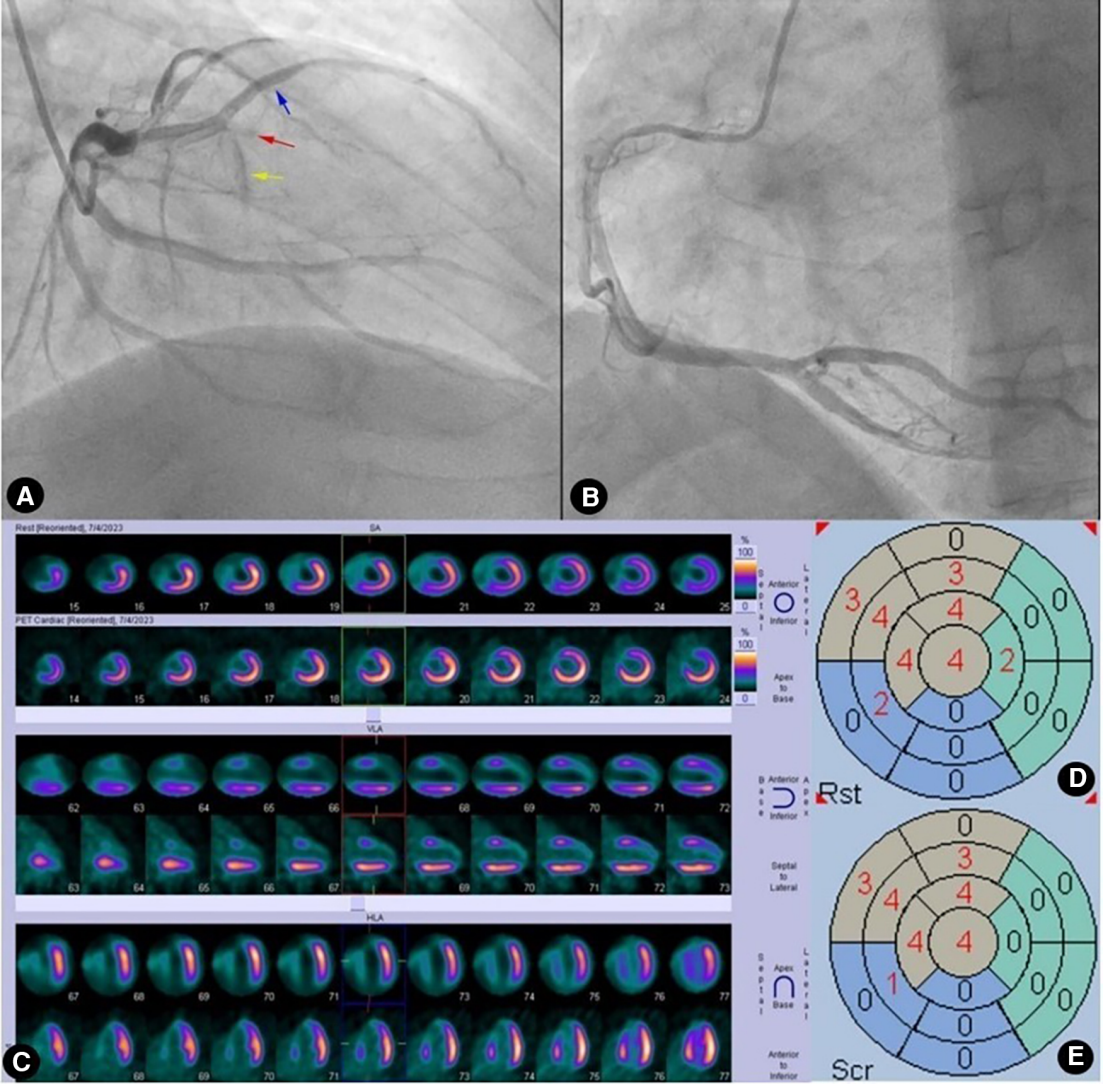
Case 1: male, 60 years old. (A) Coronary angiography showed that the left anterior descending artery (LAD) was completely occluded (red arrow) after emitting a diagonal branch (blue arrow) and septal branches (yellow arrow). (B) Coronary angiography showed no evidence of collateral circulation. (C) Rows 1, 3, and 5 presented ^99m^Tc-MIBI SPECT MPI, demonstrating segmental radiotracer deficits/defects in parts of the anterior wall, septal wall, and apex (six myocardial segments supplied by the LAD). Rows 2, 4, and 6 presented ^18^F-FDG PET MMI. The segments showing radiotracer deficits/defects in the perfusion imaging exhibited no radiotracer uptake in the metabolic imaging, indicating a perfusion/metabolism “match”. (D) Scoring for myocardial perfusion imaging. (E) Scoring for myocardial metabolism imaging. After calculations, the HM index is (22-22)/(6 × 4) × 100%=0.
